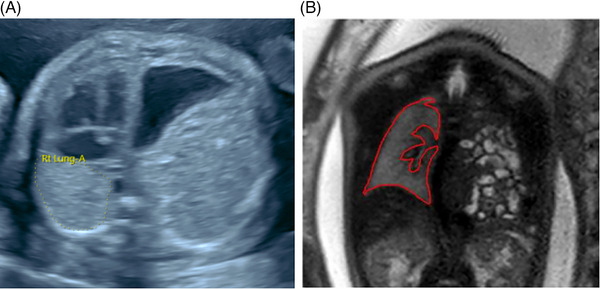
A 34‐week gestational age fetus with left‐sided congenital diaphragmatic hernia. (A) Axial ultrasound image of the fetal chest at the level of the four‐chamber heart view showing measurement of the contralateral lung via the trace method. (B) Coronal T2‐weighted single‐shot spin echo image in the same fetus showing how fetal lungs are contoured by magnetic resonance imaging (MRI) to obtain volumes.
